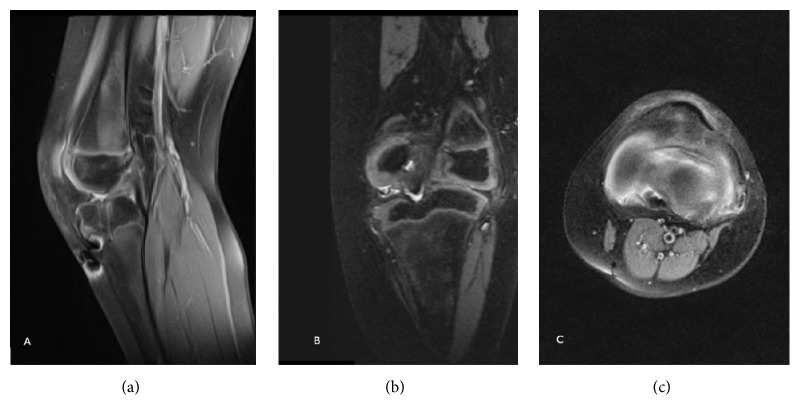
Postoperative MRI (sagittal (a), coronal (b), and transverse (c) views) of the right knee of the patient from Figures 1–4. Graft integrity was inconclusive.
